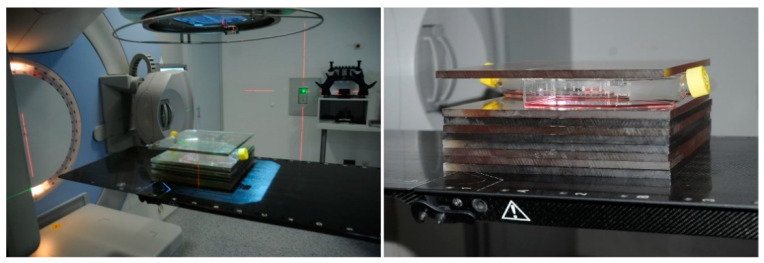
Experimental set-up for irradiation of mesenchymal stromal cells (MSC). MSC flasks were placed on 8 × 1 cm plexiglass sheets on the patient table of a Versa HD linear accelerator (Elekta). An additional 1 cm plexiglass sheet was put on top of the flasks. The laser system was used for positioning the flasks in the radiation field. All MSC in the flasks were irradiated with 2 Gy of 6 MV X-rays in orientation from top.
